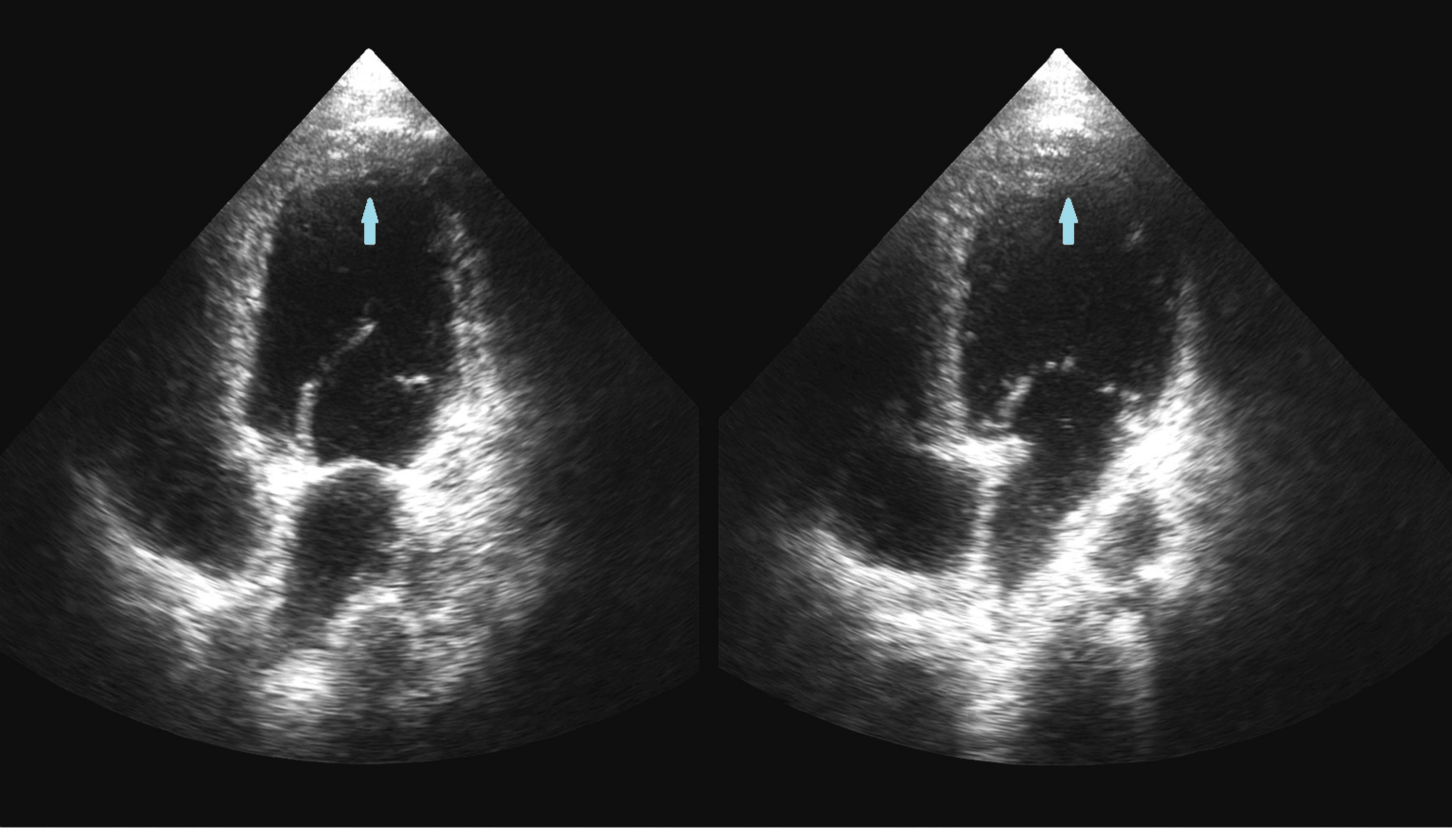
Transthoracic echocardiography (apical four-chamber view) showing apical ballooning (arrow)
